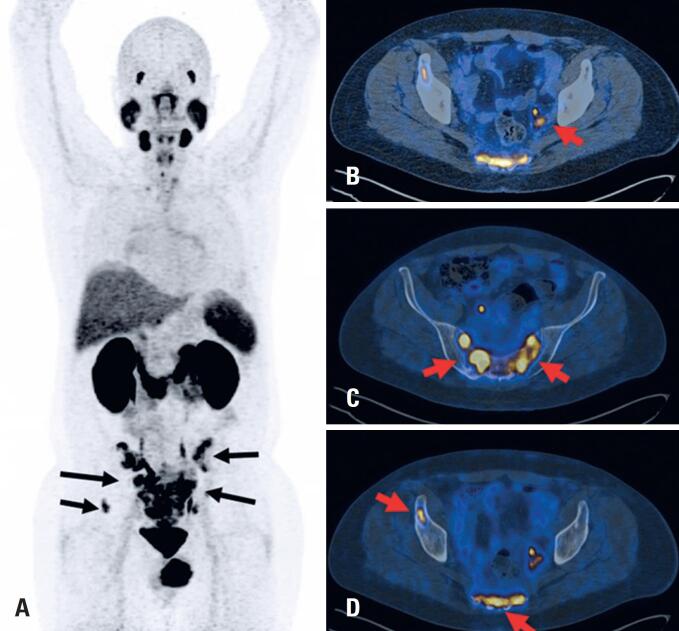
[^68^Ga]Ga-PSMA-11 PET/CT of a 62-year-old man with metastatic prostate adenocarcinoma. Whole-body PET/CT images (Biograph mCT scanner, Siemens Medical Solutions, Germany) were acquired for 4 min per bed position, 1 h after the injection of 270 MBq [^68^Ga]Ga-PSMA-11. (A) The maximum intensity projection (anterior view) shows foci of abnormal tracer uptake in multiple bone metastases in the pelvic bone. (B), (C) and (D) Fused PET/CT axial slices of the pelvis show tracer uptake in a lymph node metastasis in the left internal iliac chain (B), and bone metastases in the sacrum and right iliac bone (C and D)
